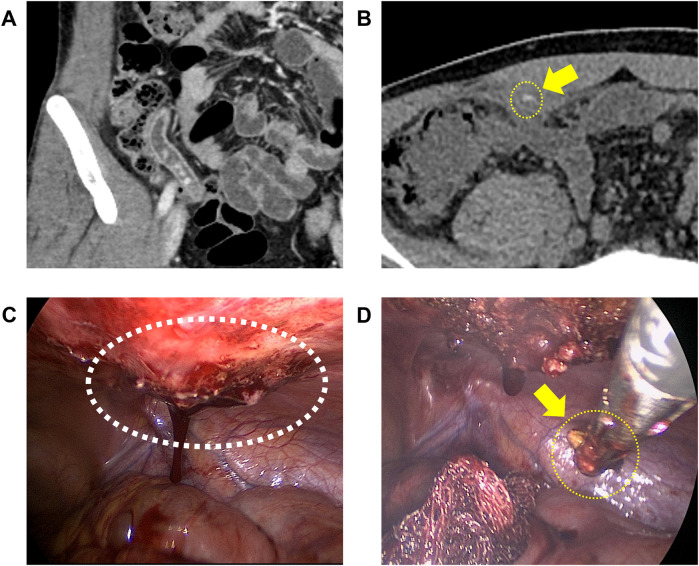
The patient who underwent reoperation. The yellow arrow indicates the dropped appendicolith. (A) Multiple appendicoliths were identified before the initial appendectomy. (B) CT on postoperative day 20 revealed a 20 mm intra-abdominal abscess with a 2 mm appendicolith inside. (C) Findings of laparoscopic view during reoperation. The white circle indicates an intra-abdominal abscess surrounded by granulation tissue at the abdominal wall. (D) Removing appendicolith after the excision of granulation tissue.
